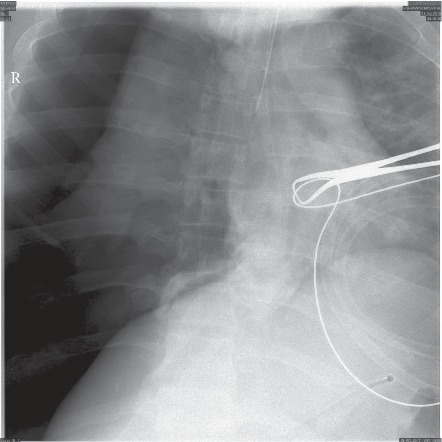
Intraoperative chest radiograph showing right-sided pneumothorax.
